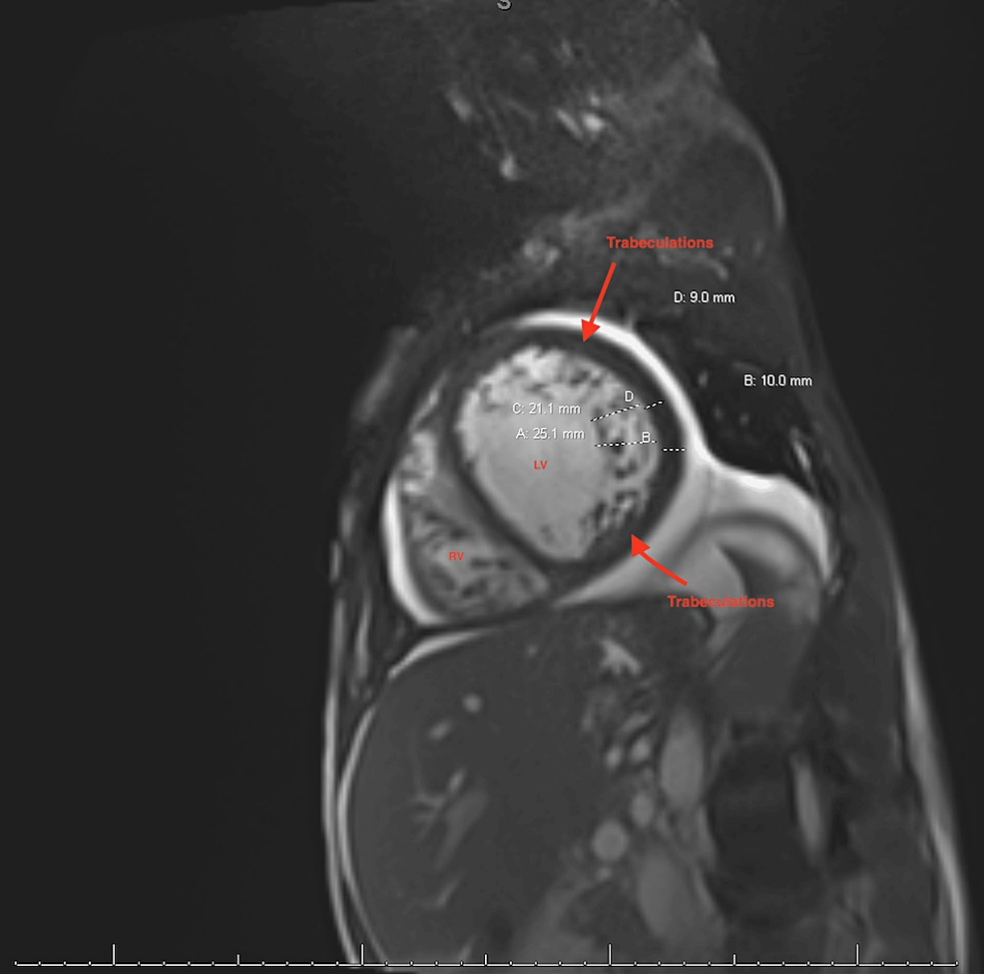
Cardiac MRI (sagittal view) with hypertrabeculations meeting the criteria for LVNC cardiomyopathy LVNC: Left ventricular non-compaction
